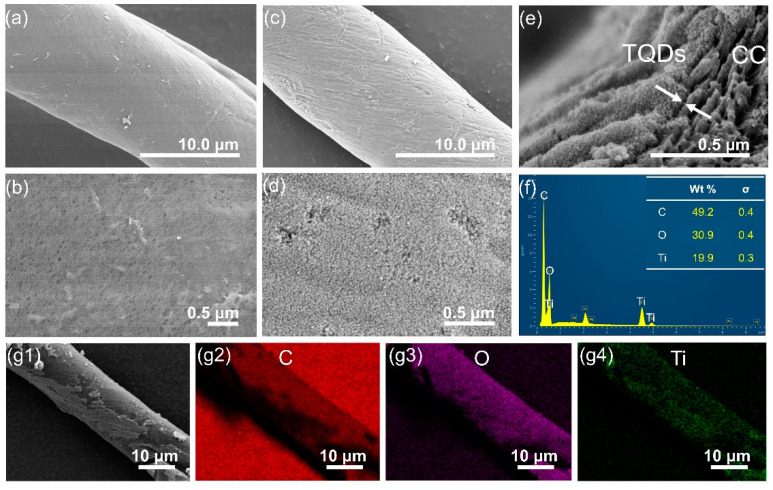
(a,b) SEM images of CC. (c–e) SEM images and corresponding elemental analysis (f) and SEM-EDX mapping results after an ultrasonic treatment (g1–g4) of the TQDs/CC-2.5 sample.
